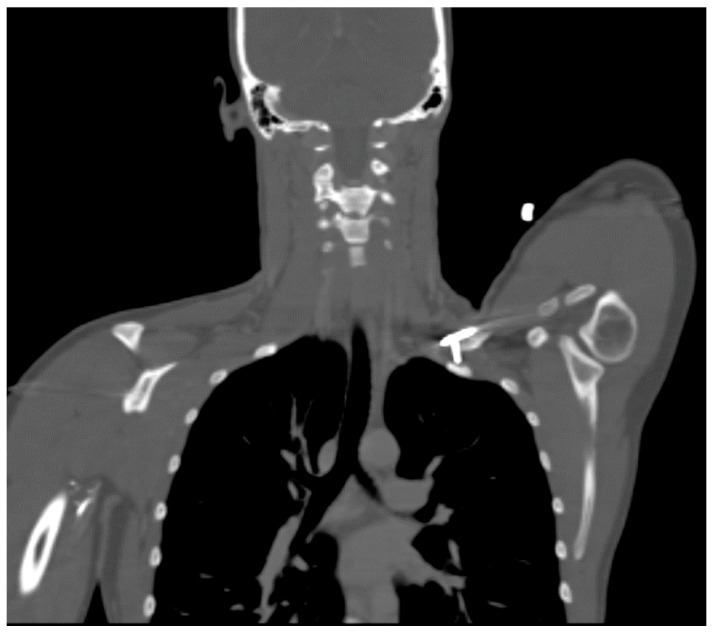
CT angiogram demonstrating a screw abutting the thoracic outlet in an ATOS patient with a subclavian artery aneurysm.
